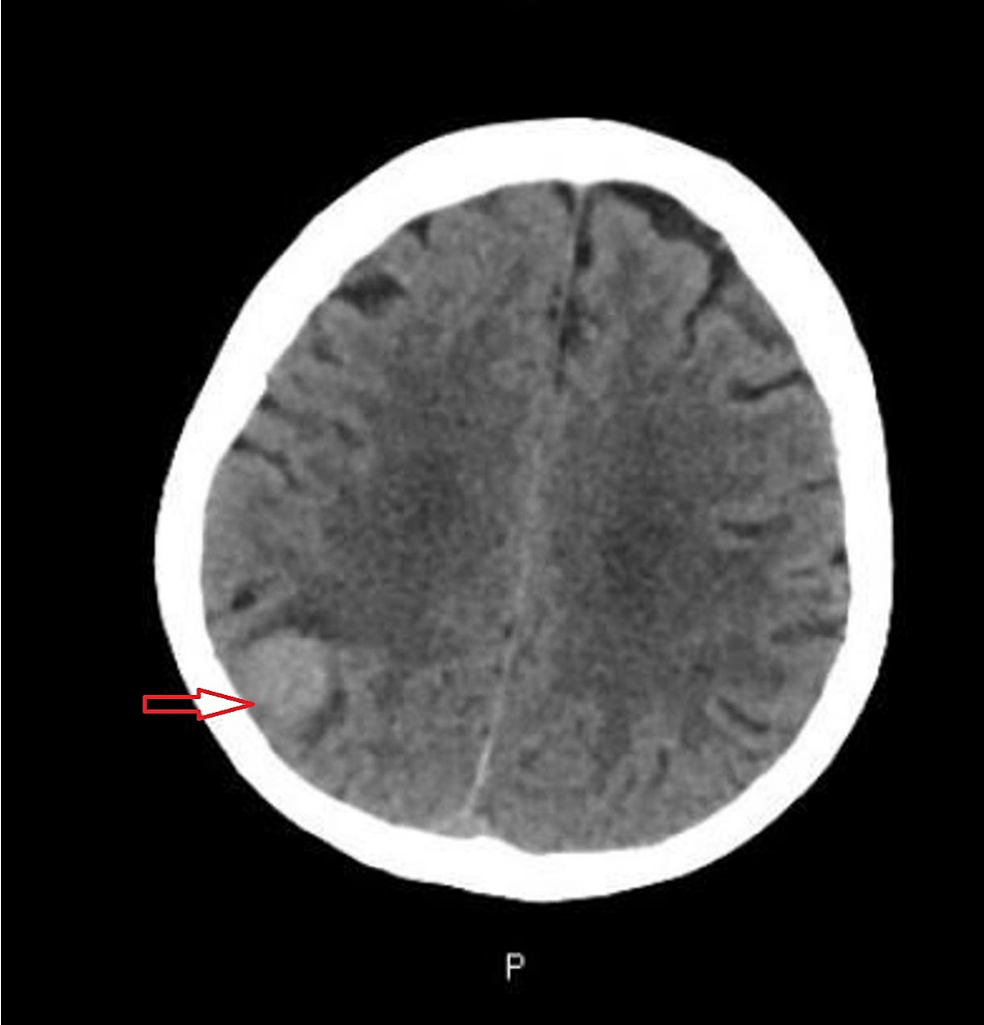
CT Head (Axial View) Showing Hyperdense Lesion in the Right Posterior Parietal Lobe
